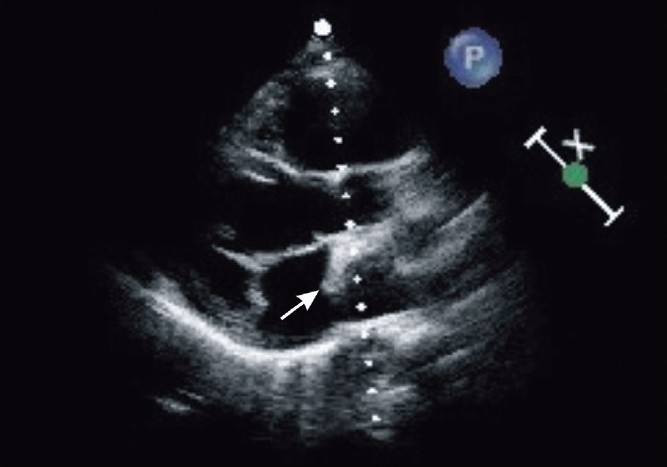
Transthoracic 2D echo: parasternal long axis view showing Cor–triatriatum
